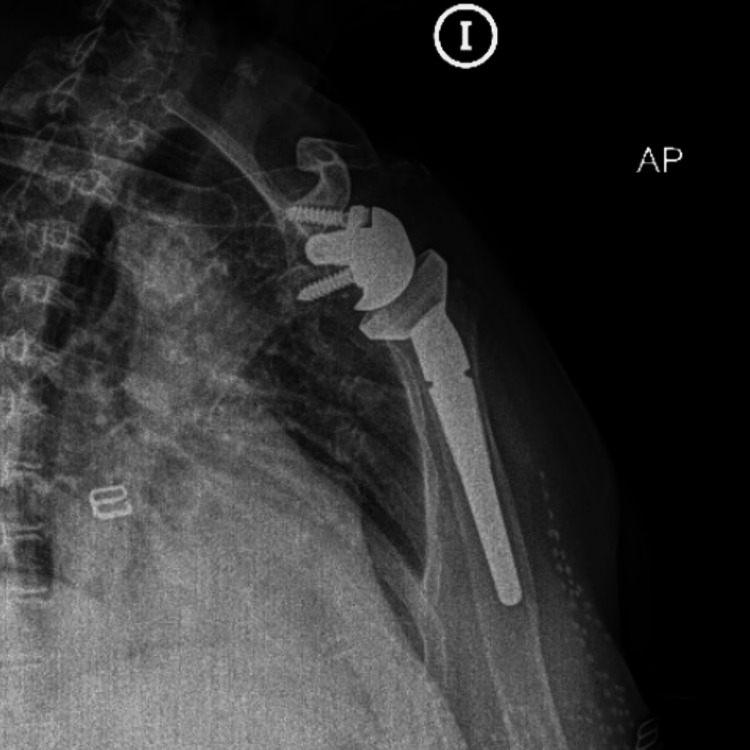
Post-surgical radiograph showing the presence of an uncemented reverse right shoulder prosthesis.
